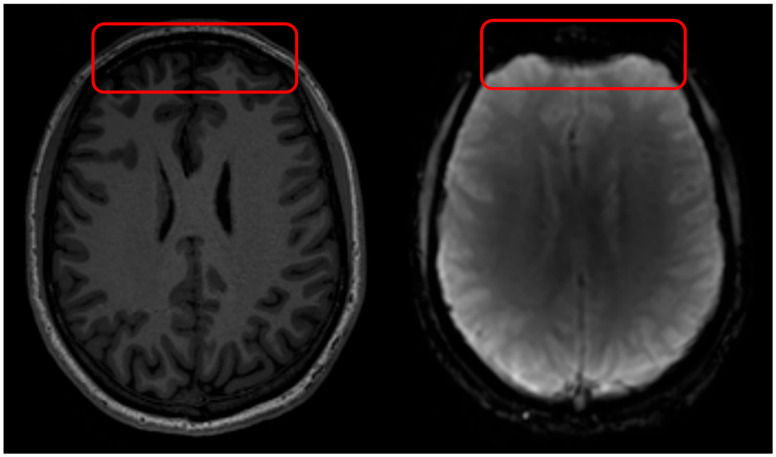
Geometric distortions in DSC MRI appear as misshapen or incorrectly sized brain structures, leading to spatial misregistration with anatomical images, primarily in regions near air/tissue interfaces. Geometric distortions in DSC MRI arise due to B_0_ field inhomogeneities and tissue susceptibility differences. On the left is a T_1_w MRI scan and on the right a DSC MRI scan with visible geometric distortions. The red square indicates tissue hyper intensities due to B_1_ field inhomogeneities.
